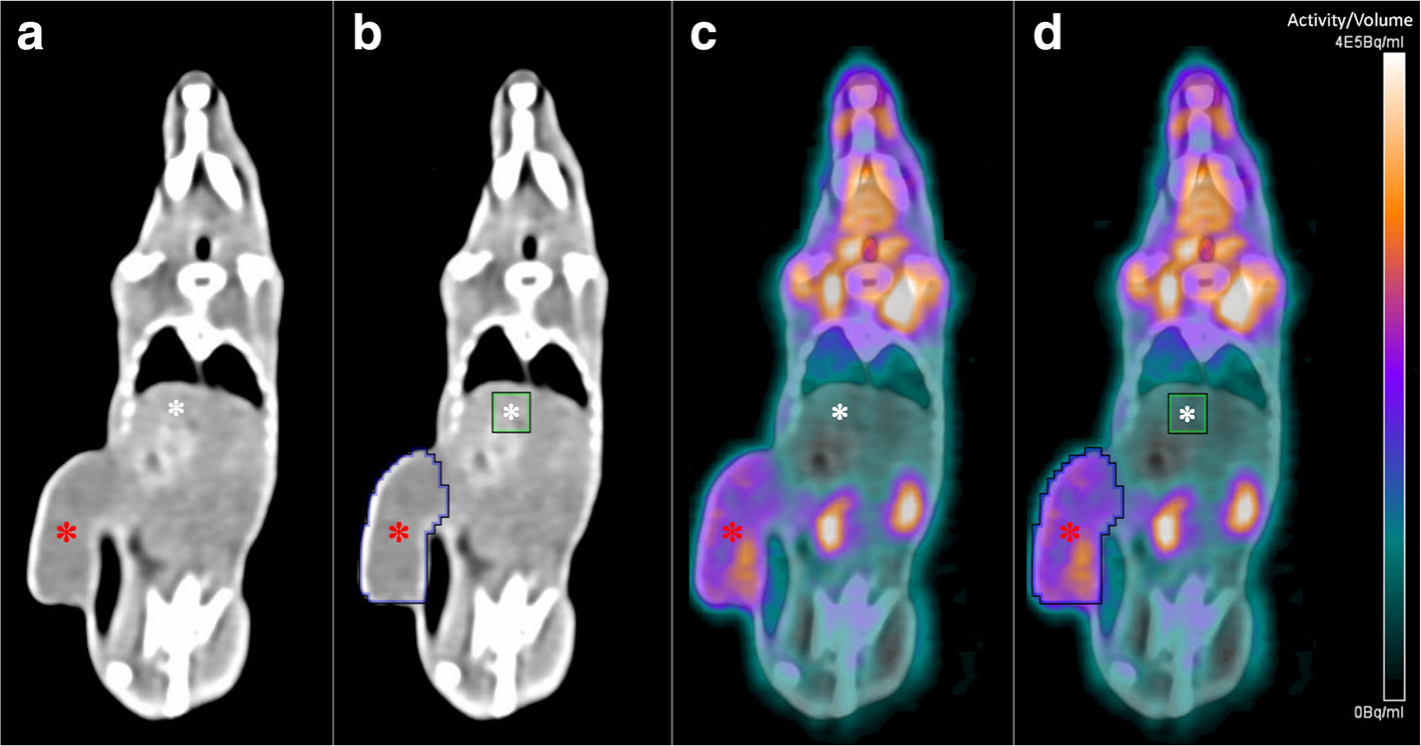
PET/CT VOI Selection. a and b: coronal unenhanced CT without (a) and with (b) VOIs. c and d: Fused coronal PET/CT data sets without (c) and with (d) VOIs. Note the tumor in the lateral flank (red asterisk). The liver is indicated by a white asterisk. To calculate the metabolic tumor volume, a PET/CT-guided VOI surrounding the tumor was drawn. Background was determined in a VOI in the right liver lobe and the TTL was calculated accordingly
